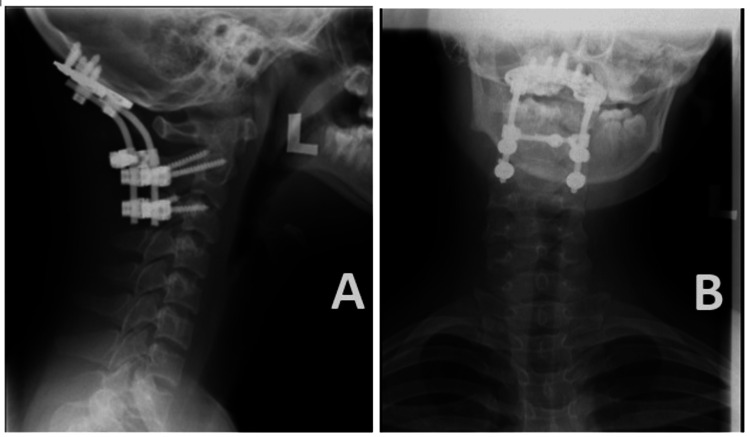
One-year follow-up cervical radiograph revealed that implants were in situ with no signs of loosening and the cranio-cervical alignment was well maintained. Lateral view (A) and anteroposterior view (B) of the cervical radiograph.
